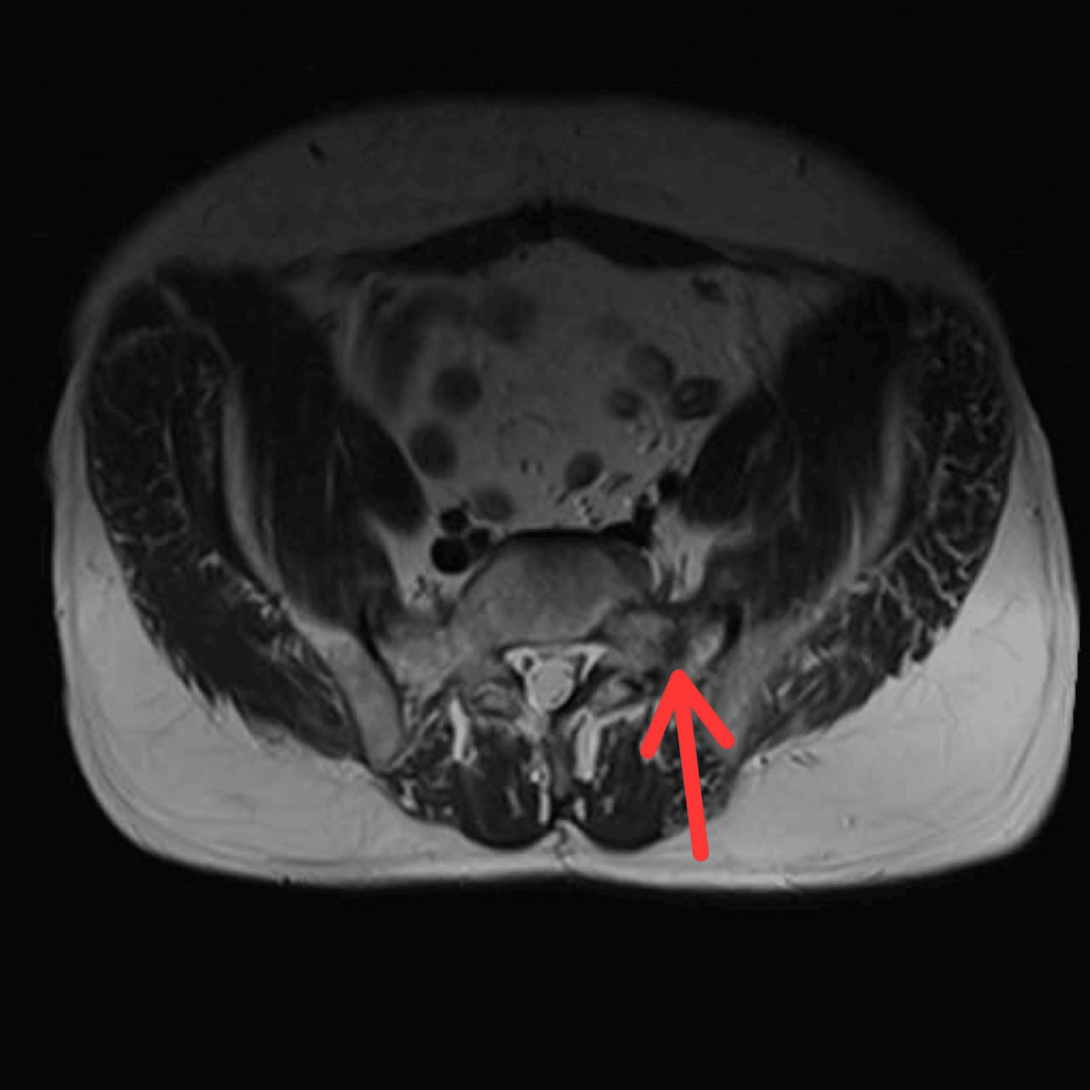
MRI showing a non-displaced fracture of the left sacral ala (red arrow) after radiotherapy to the rectum for rectal cancer. The examination was performed on October 3, 2022, in the radiology department at Bolton NHS Trust, UK.
